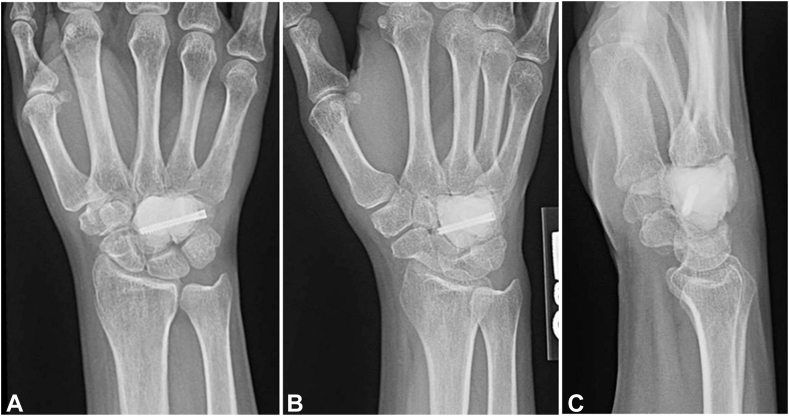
A–C Plain radiographs 15 months after surgery from cemented capitohamate fusion.
